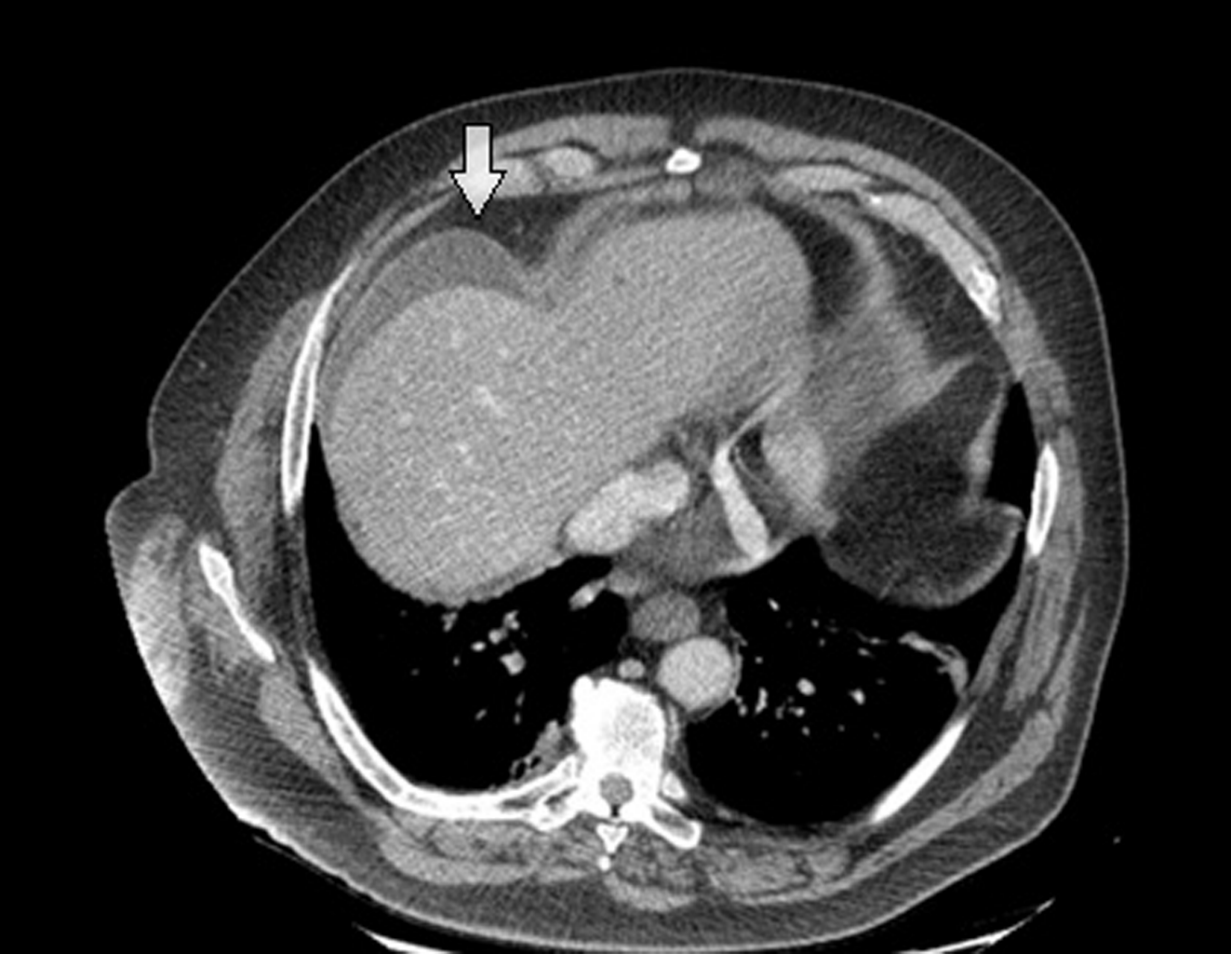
CT showed intraperitoneal free fluid.
